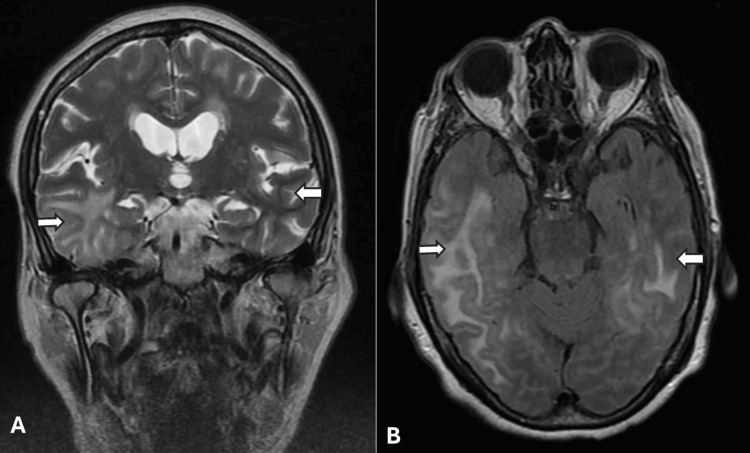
Initial MRI of the brain T2 coronal (A) and T2 FLAIR (B) images demonstrating extensive white matter hyperintensities and vasogenic edema throughout bilateral temporal lobes, the right side greater than the left side. MRI: magnetic resonance imaging; FLAIR: fluid-attenuated inversion recovery
